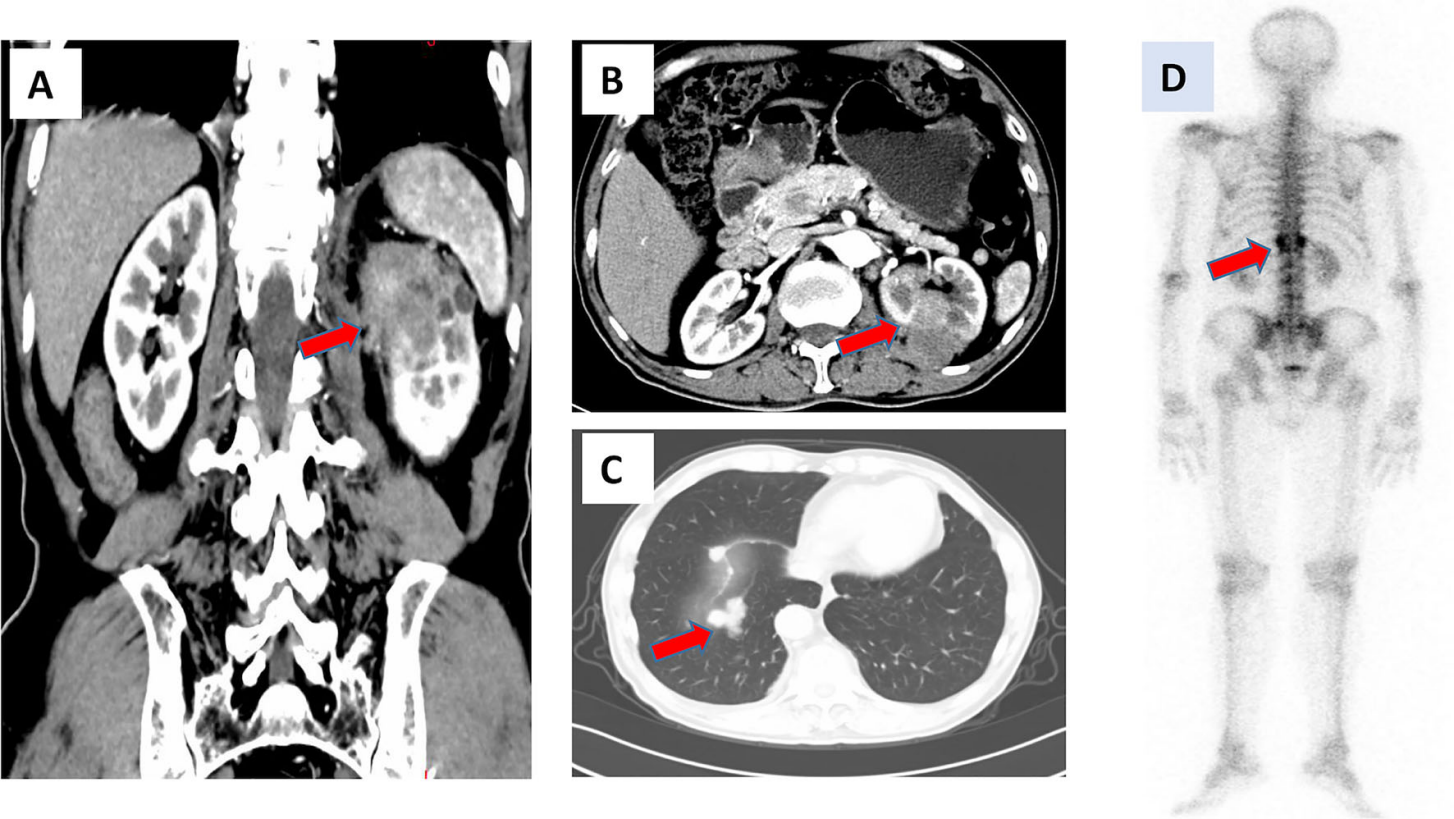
Radiological examination at initial visit. (A, B) Contrast-enhanced CT scan of the abdomen showing a malignant mass in the left kidney. (C) Chest CT scan showing metastatic nodules in the right lower lung. (D) Whole body bone scan showing abnormal T12 vertebration. CT, computed tomography.
